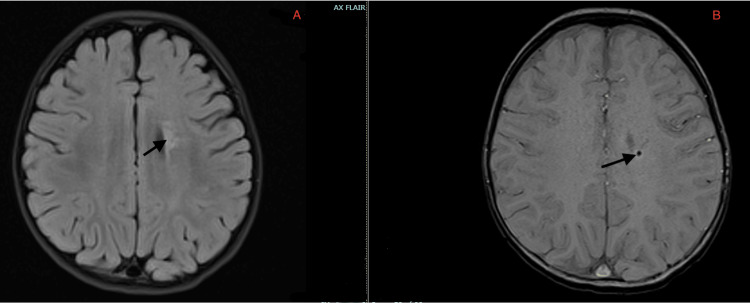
MRI brain (A) Arrow shows a very small left periventricular FLAIR change (bright spot); (B) Arrow shows a small amount of hemosiderin deposition. FLAIR: Fluid-Attenuated Inversion Recovery; MRI: Magnetic Resonance Imaging
